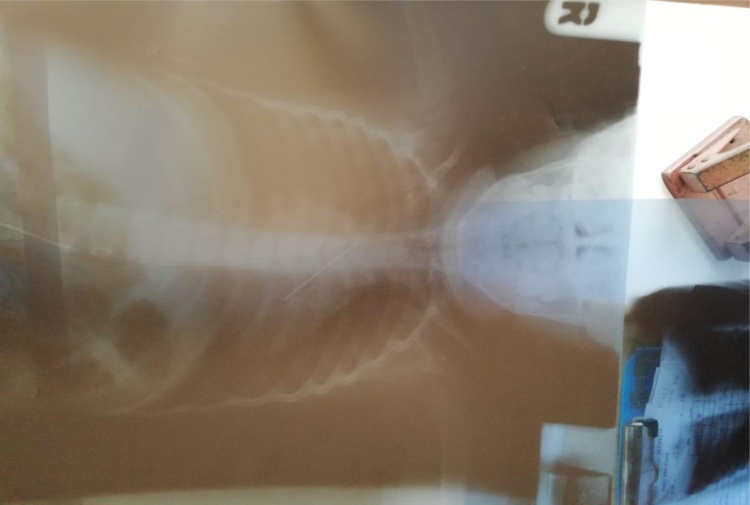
Chest x-ray showing the metallic pin being located in the left main bronchus.
